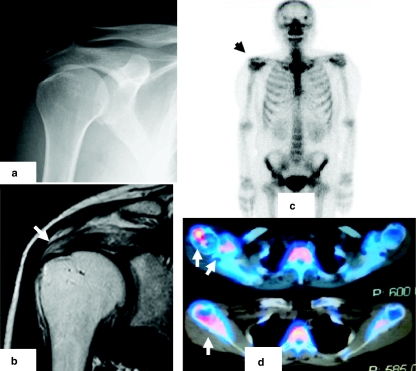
Case 2: A 51-year-old male with a rotator cuff tear in the right shoulder. No obvious osteoarthritis is observed in X-rays (A). A full-thickness rotator cuff tear was observed on the coronal T2 WI MRI (B, arrow). An increase of RI uptake in the left shoulder joint is observed in the bone scintigram (C, arrow). In SPECT images, RI uptakes are evident in the greater tuberosity of the humerus, the coracoid process of the scapula, and the glenohumeral joint (D, arrows).
